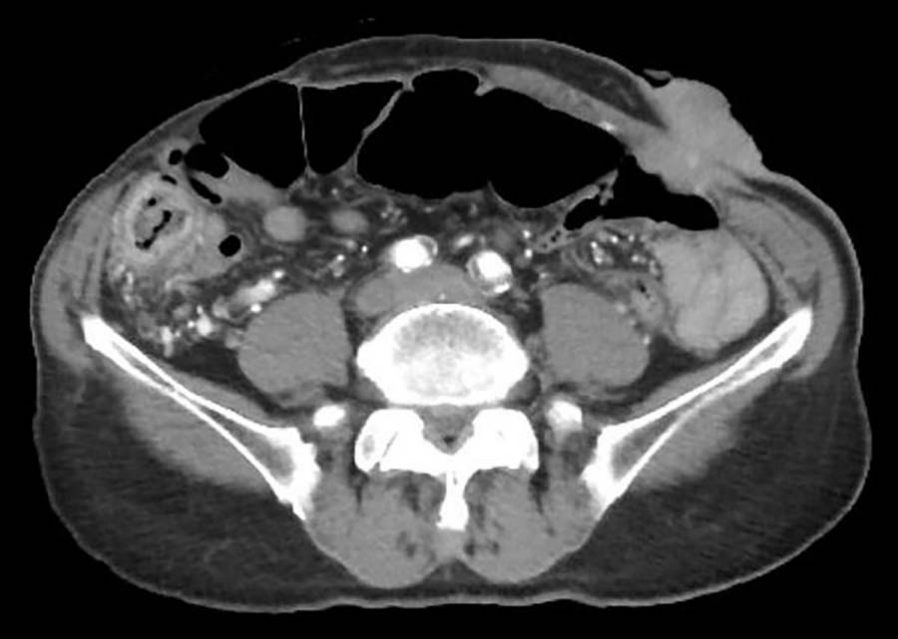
Computed tomography showed irregular mass at the abdominal wall.
